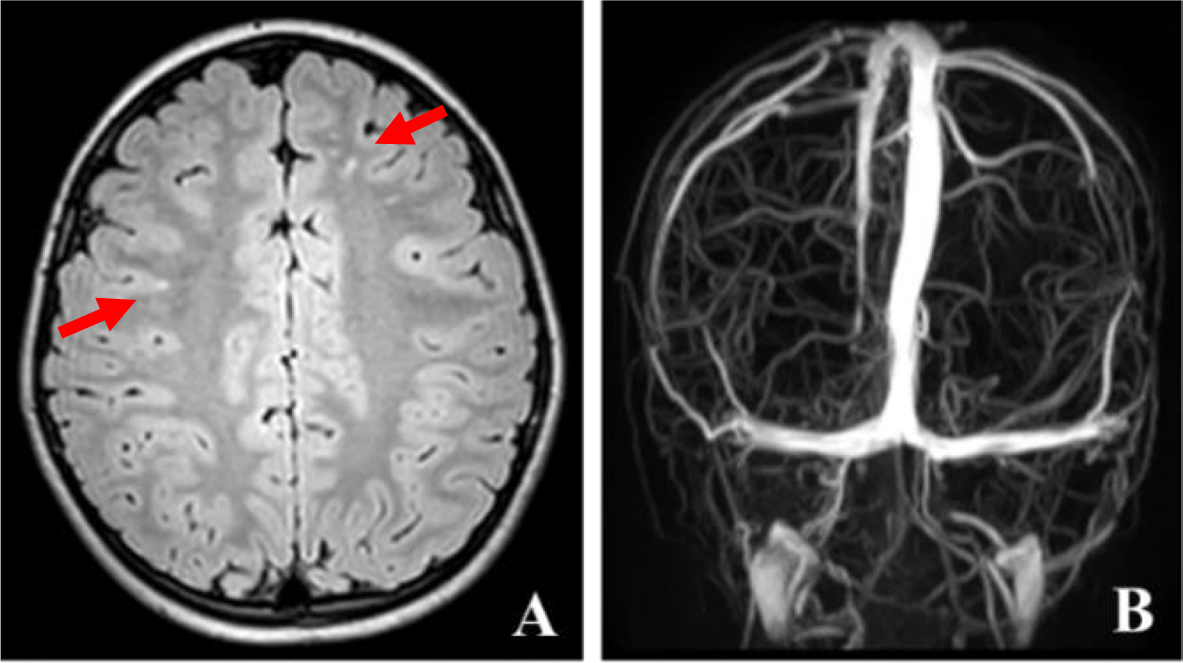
Representative scanning images of the nervous system. Panel A: MRI image of the patient's brain. Deep left frontal lobe and the right parietal lobe showing high signal intensity at T2 FLAIR imaging, as indicated by the arrows. Panel B: MRV image of the patient's brain without noticeable abnormalities. MRI, magnetic resonance imaging; MRV, magnetic resonance venography
